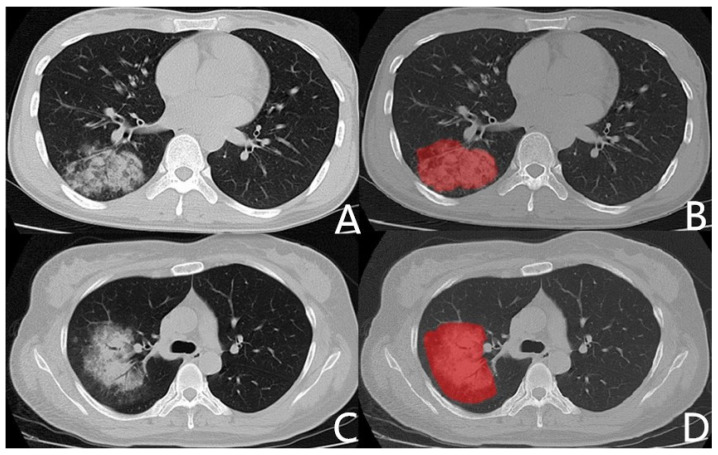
Chest CT images and related ROIs. (A): Chest CT of mycoplasma pneumonia. Male, 27 years old, fever for 6 days, maximum temperature 39.8 °C, CRP 73 mg/L, WBC 4.9 × 10^9^/L, neutrophil 67.7%. (C): Chest CT of bacterial pneumonia (pseudomonas aeruginosa). Female, 39 years old, fever for 5 days, maximum temperature 37.5 °C, CRP 12 mg/L, WBC 9.8 × 10^9^/L, neutrophil 65.1%. (B,D): ROI delineated with ITK-SNAP.
